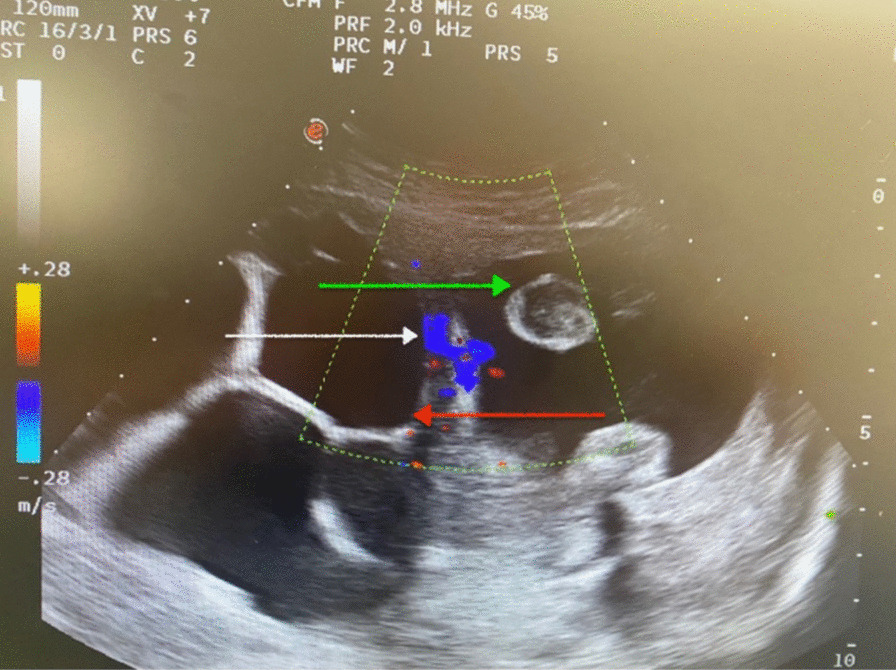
Abdominal ultrasound showed a multilocular cyst at the right side of the uterus (red arrow) with intense vascularized on Doppler ultrasound (white arrow). Thin uterine wall with fetal parts (green arrow)
